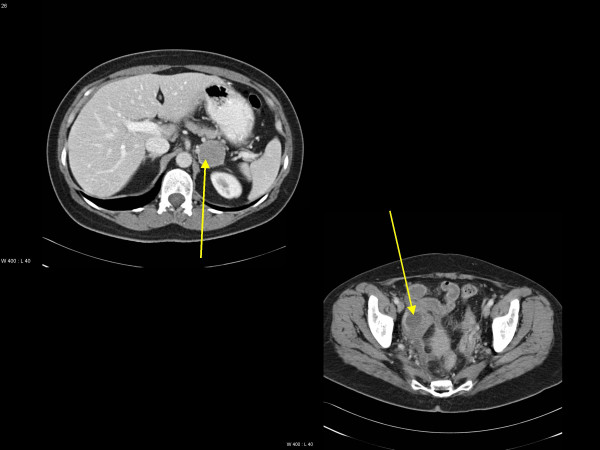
Computed tomography scans of the abdomen. Adrenal gland metastasis (upper scan) and lower abdominal mass resulting from inflammation around the melanoma in the vermiform appendix 4 months later (lower scan).
